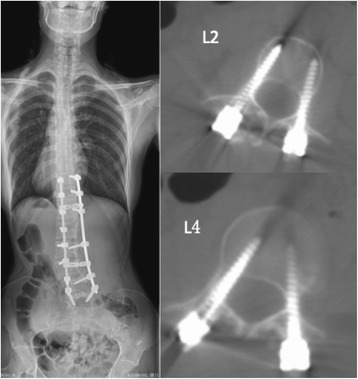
Postoperative CT scanning demonstrating thin pedicle could not contain screw in L2 level and medial perforation in L4 level
